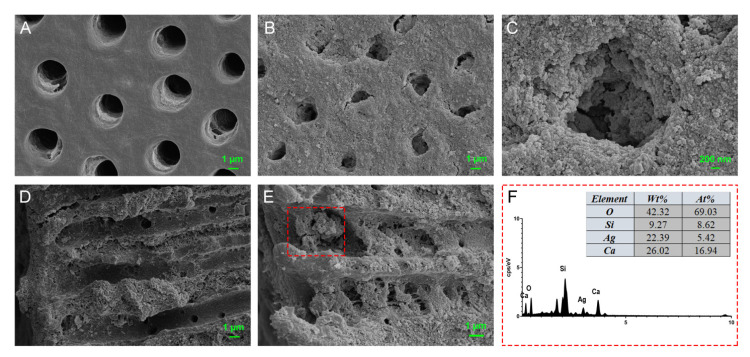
Infiltration of M-AgTX into dentinal tubules. (A) Original dentinal tubule openings (A) ×5000; (B,C) Tubule openings treated ultrasonically with M-AgTX (B) ×5000, (C) ×20,000); (D) Original dentinal tubule axial cross sections (D) ×5000; (E) Tubule axial cross sections after being treated with M-AgTX (E) ×7000; (F) EDS spectrum of selected square area in (E).
